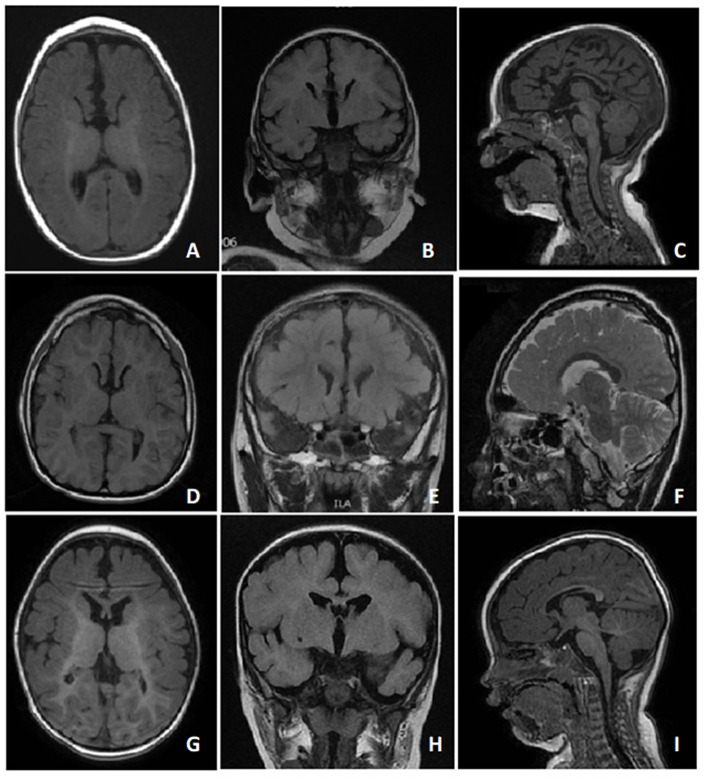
T1 and T2 MRI images of case 1 (A-C) at 6 months of age, case 2 (D-F) at 7 years of age, and case 3 (G-I) at 11 months of age showed dysgenesis of the corpus callosum, including the genu and body portions of the corpus callosum.
